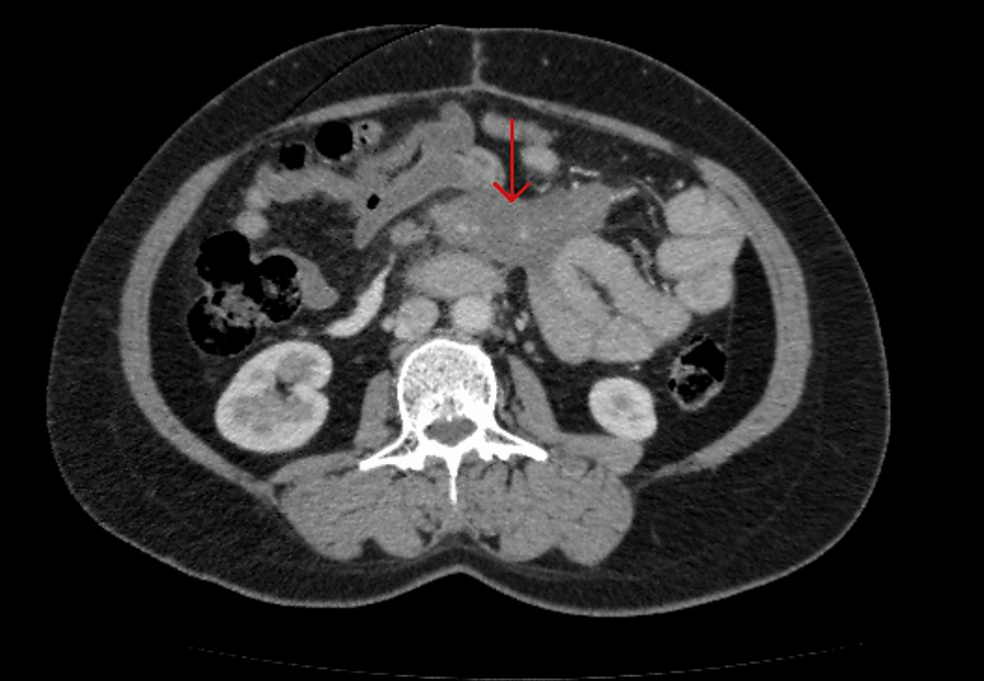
CT scan showing the mesenteric mass encasing the mesenteric artery. A CT scan at the six-month follow-up showing a mild regression in mass size, compared with Figure 1. Red arrow pointing to the mass.
